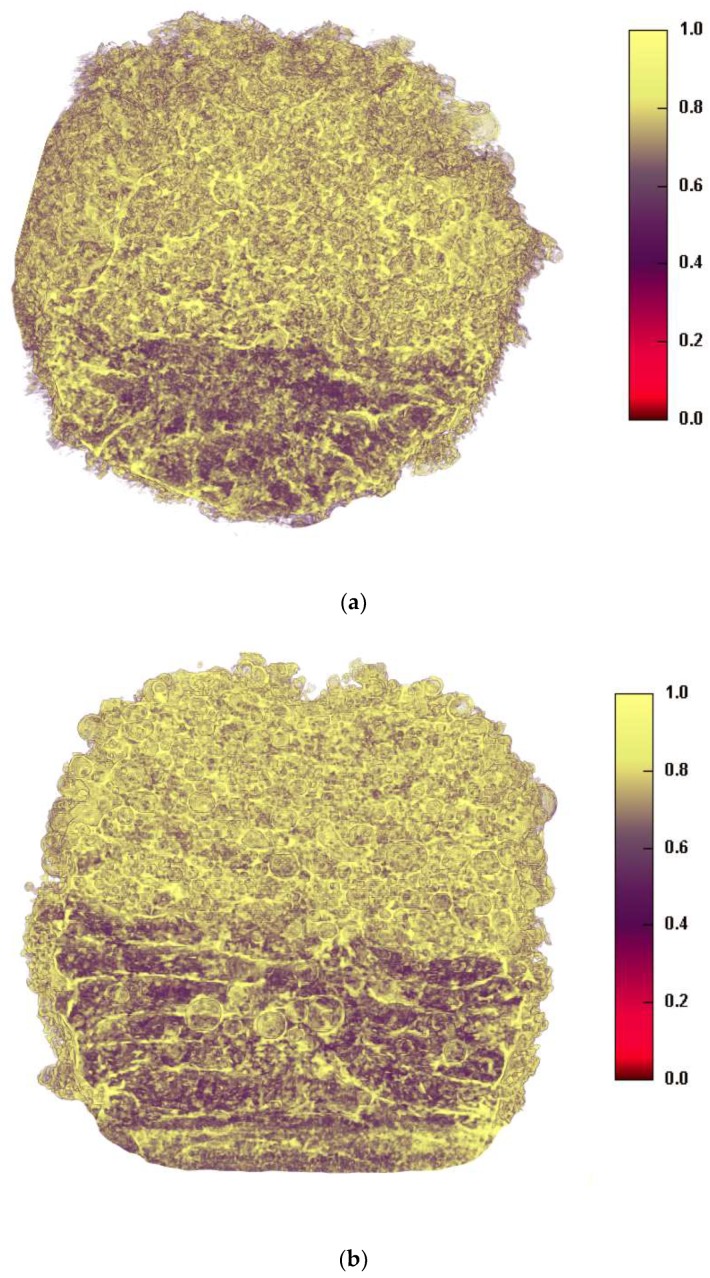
Cross-sectional x-ray micro computed tomography (CT) images of (a) Con A and (b) Con B of the 2 mm dual miniprintlets. The yellow regions represent the Kollicoat Instant Release (KIR) regions, whereas the dark purple regions are the ethyl cellulose (EC) regions. The scale bar is representative of density.
